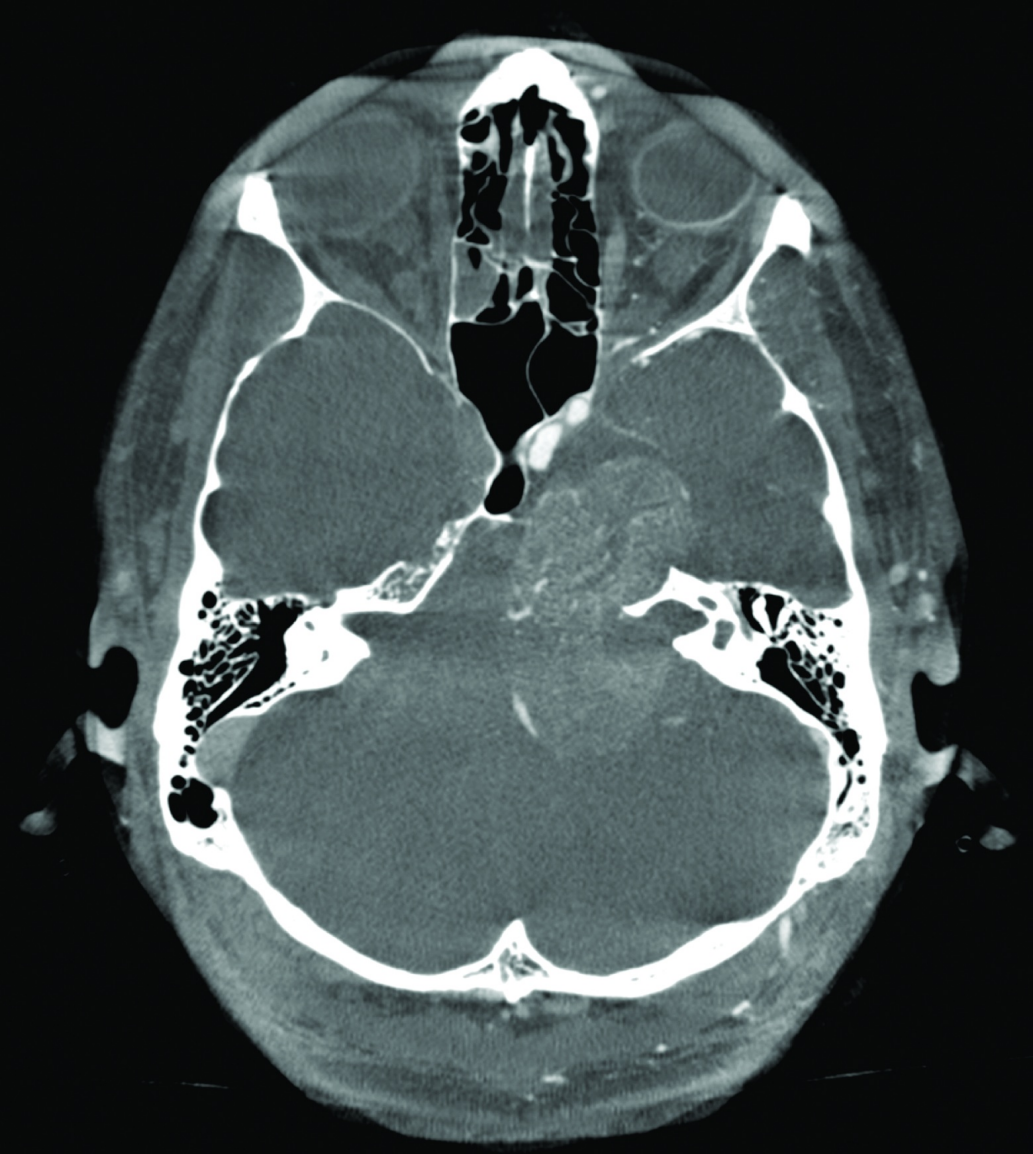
Preoperative CT scan Axial computed tomography (CT) scan (bone windows) showing a lesion at the level of the left Meckel´s cave with significant petrous bone remodeling.
